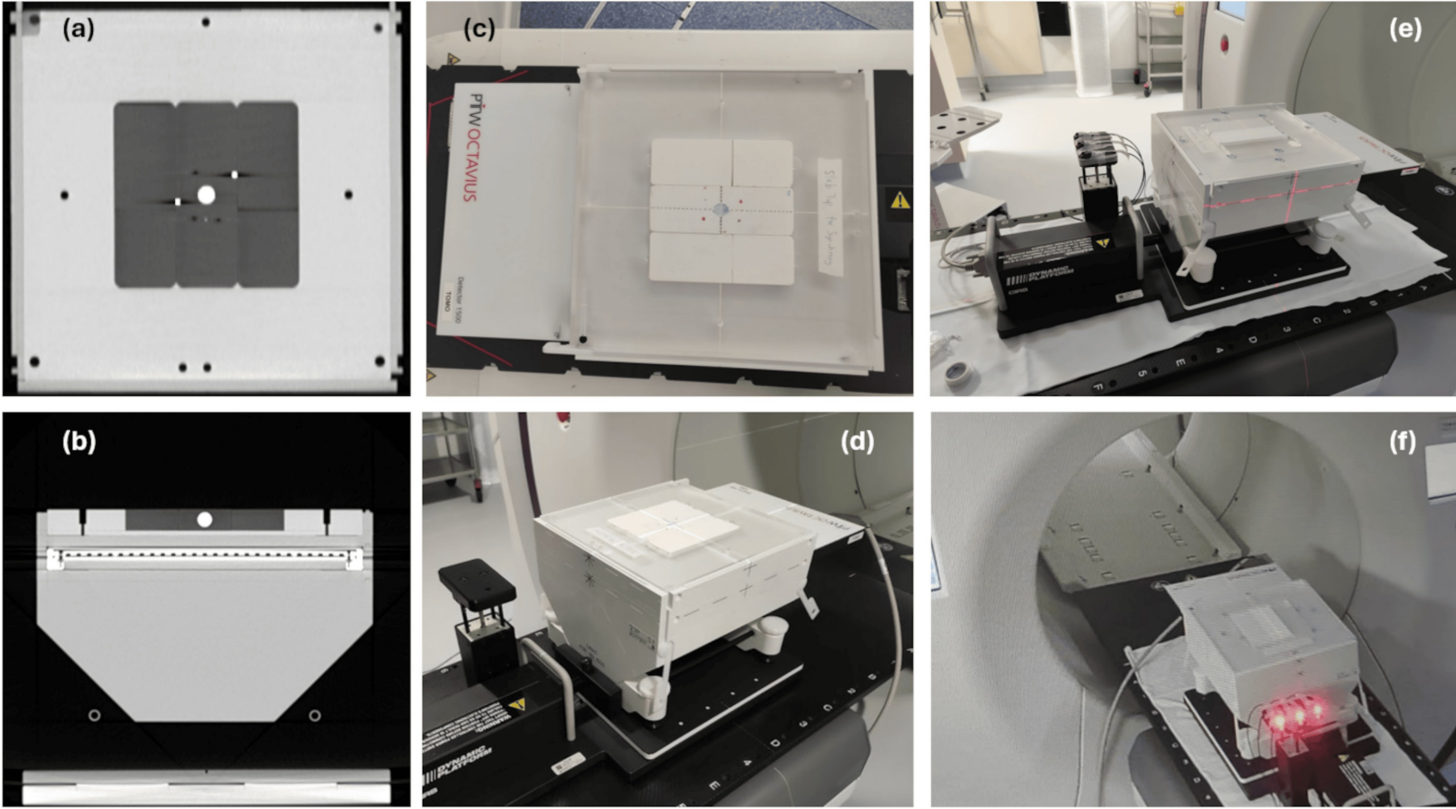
CT images and photographs of the dynamic QA system setup for Synchrony verification. Dynamic QA system as shown in (a) coronal and (b) transverse slices through the CT scan, (c) and (d) photographs of the Synchrony fiducial tracking verification setup, and (e) and (f) photographs of Synchrony lung tracking with the respiratory modelling verification setup.
